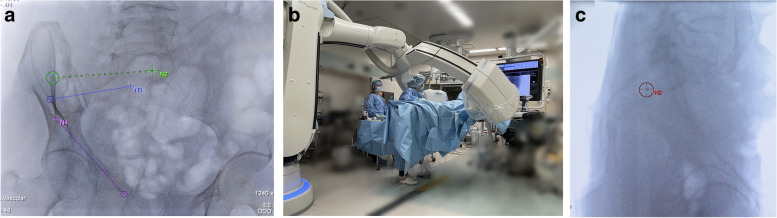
Real-time 3D fluoroscopic navigation. The virtual trajectory was projected and overlayed onto the real-time fluoroscopic images and displayed on a dedicated live monitor (a). The C-arm was rotated to the “bull’s eye view” b where it was angulated in a way that the cross and the circle displayed on the live monitor completely matched and the central X-ray beam was aligned with the planned trajectory (c)
